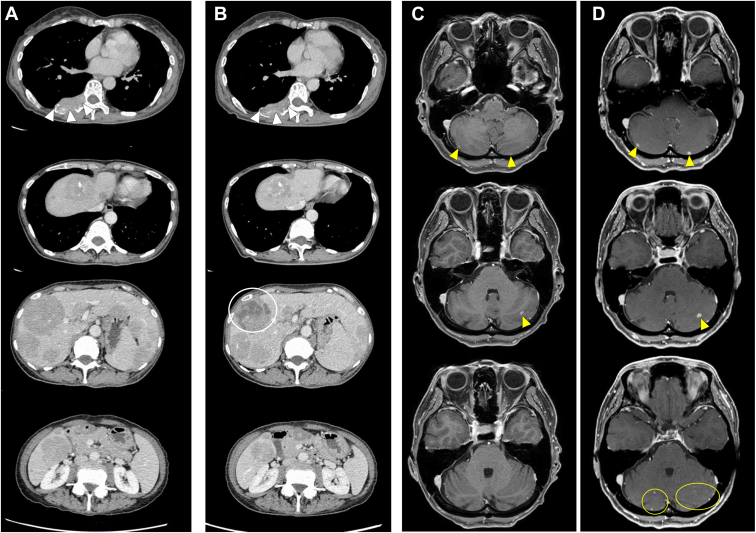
Contrast-enhanced computed tomography and brain magnetic resonance imaging before and after tarlatamab administration. Contrast-enhanced computed tomography images (*A*) before and (*B*) on day 15 after tarlatamab administration. The right inferior rib metastasis (white arrowheads) and multiple liver metastases show reduction in size. In addition, non-enhancing areas suggestive of necrosis are observed within some of the liver lesions (white circle). Brain magnetic resonance imaging (*C*) before and (*D*) on day 50 after tarlatamab administration. Enlargement of existing cerebellar metastases (yellow arrowheads) and the emergence of new multiple cerebellar metastases (yellow circle) are observed.
